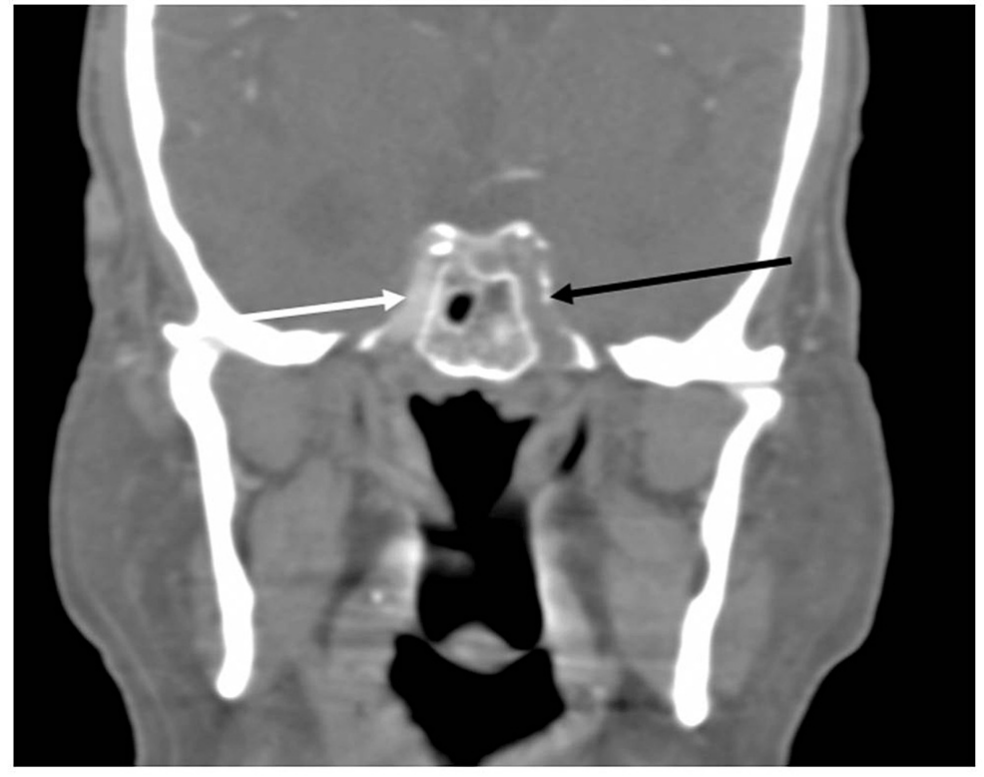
Computed tomography angiography at the level of the cavernous portion, coronal view Computed tomography angiography at the level of the cavernous portion coronal view demonstrates total occlusion of left internal carotid artery (black arrow) while the right internal carotid artery (white arrow) is opacified.
